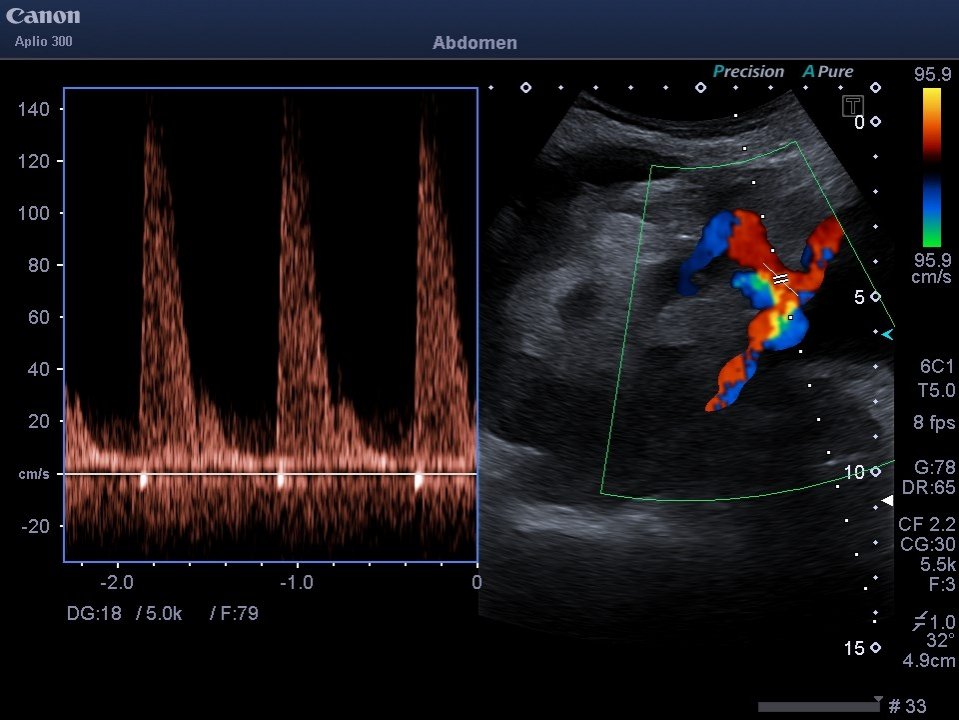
POCUS pulsed wave Doppler with aliasing at anastomosis level showing turbulent flow even when adjusted for high pulse repetition frequency to reduce noise.
